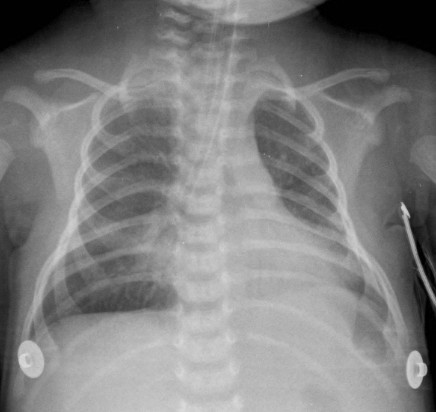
Chest X-ray after 24 hours of ILV.
